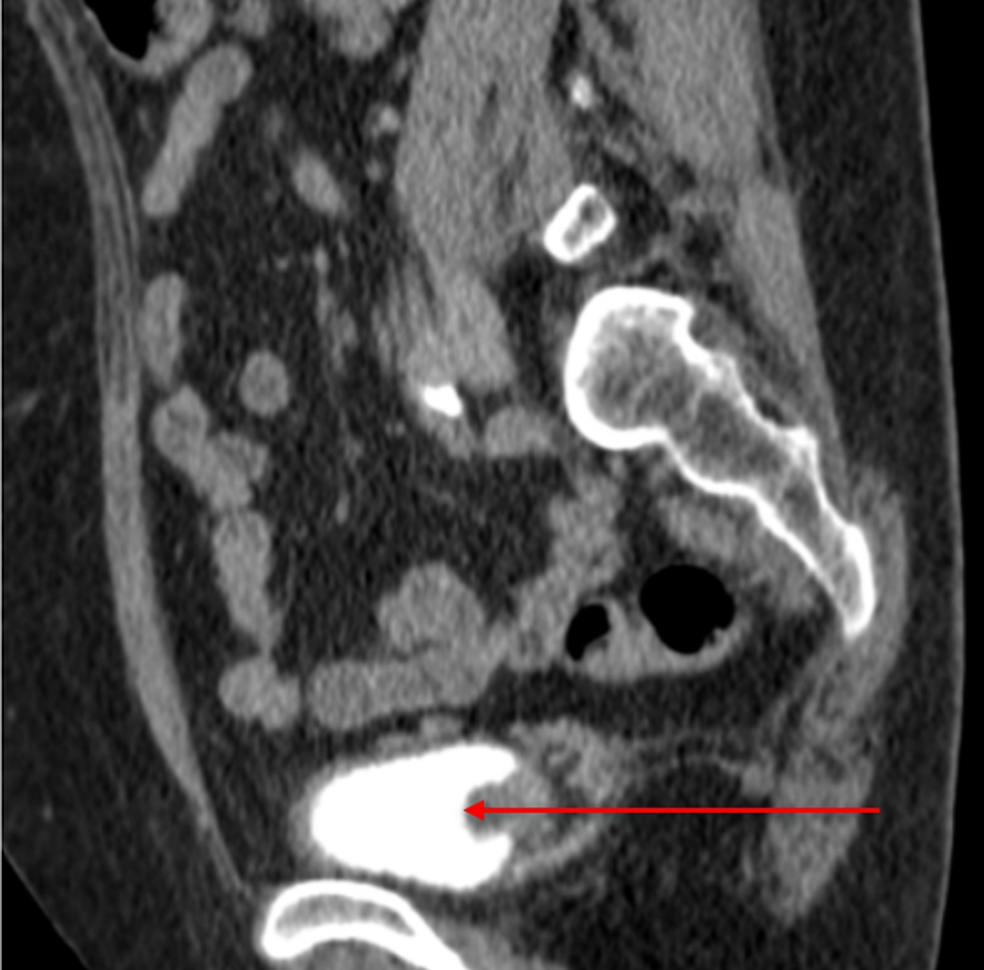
Coronal computed tomography view of the tumor in the bladder (red arrow pointing to the tumor)
